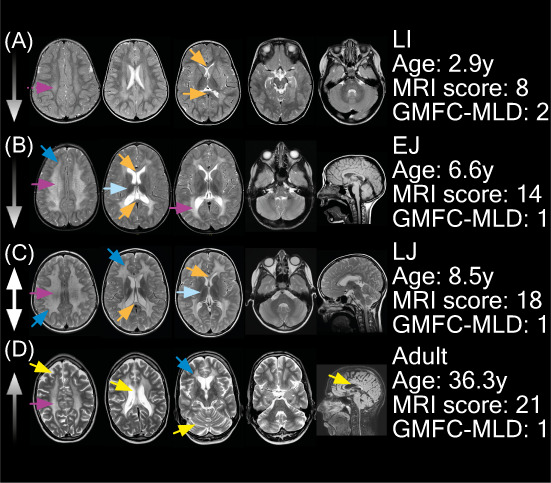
Typical MRI abnormalities in symptomatic MLD patients. Patient A had a PNS‐dominant motor phenotype, with CNS symptoms present. Patient B had a mixed phenotype with both motor and cognitive characteristics, but it was a PNS dominant phenotype. Patients C and D had a mixed phenotype, although these patients had a CNS dominant phenotype. Corpus callosum involvement; No (MLD MRI score ≤6); No (MLD MRI score >6); Yes (MLD MRI score ≤6); Yes No (MLD MRI score >6).
